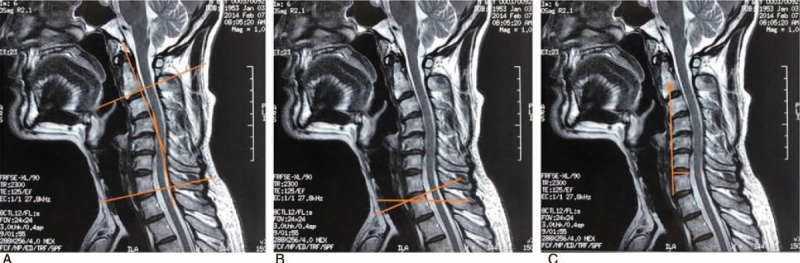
Preoperative neutral sagittal view of T2-weighted image in 2014. (A) showed C2–C7 Cobb angle; (B) showed the angle of T1 slope; (C) showed C2–C7 sagittal vertical axis.
